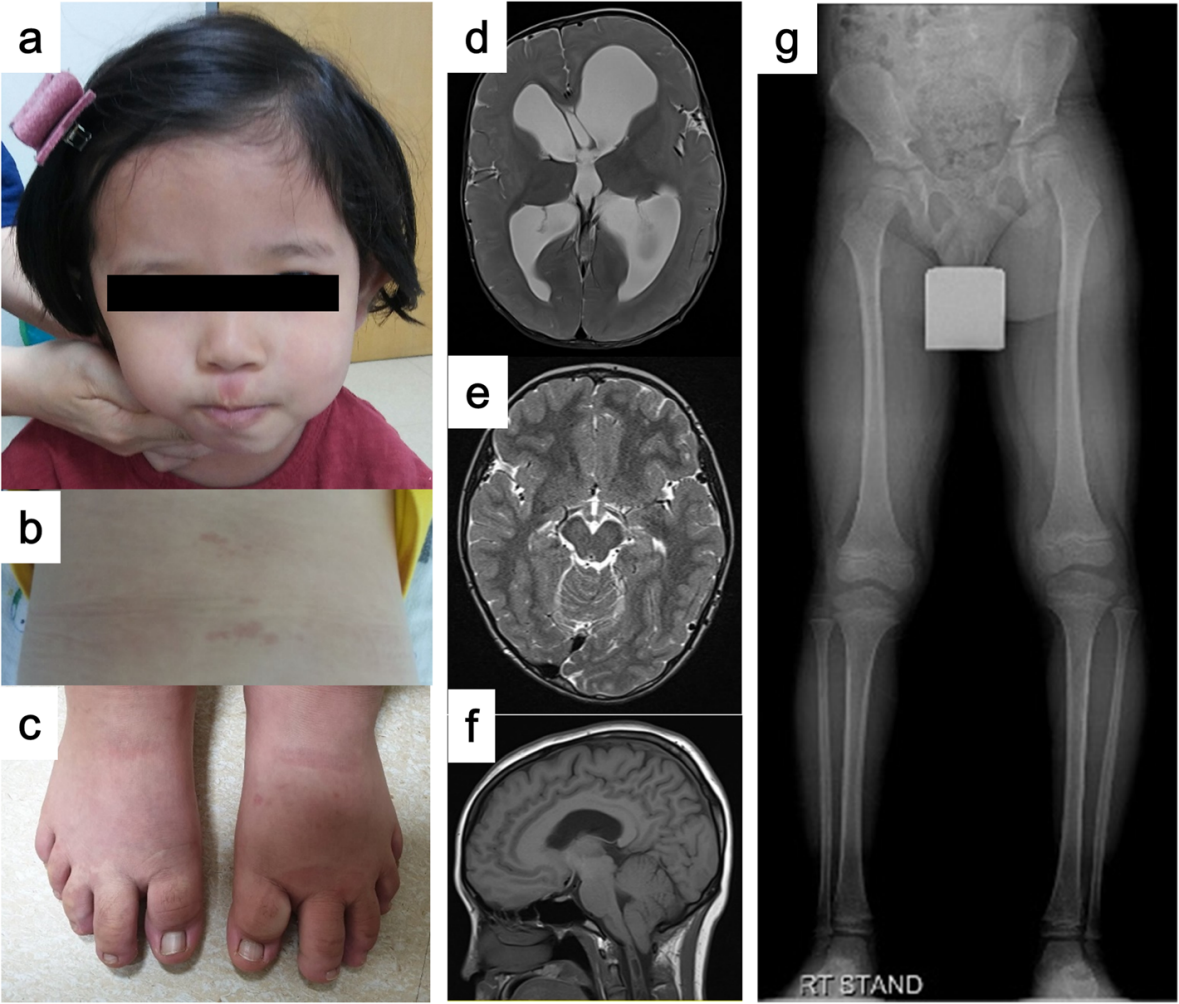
Images of the patients showing various clinical manifestations. a Frontal bossing, facial asymmetry, and cutaneous capillary malformation, hemangioma-like lesions on the philtrum. b Cutaneous capillary malformation on the back. c Hypertrophy of bilateral first and second toes. d, e Left-sided hemimegalencephaly. f Arnold–Chiari type I malformation. g Leg length discrepancy is shown in a simple radiograph
